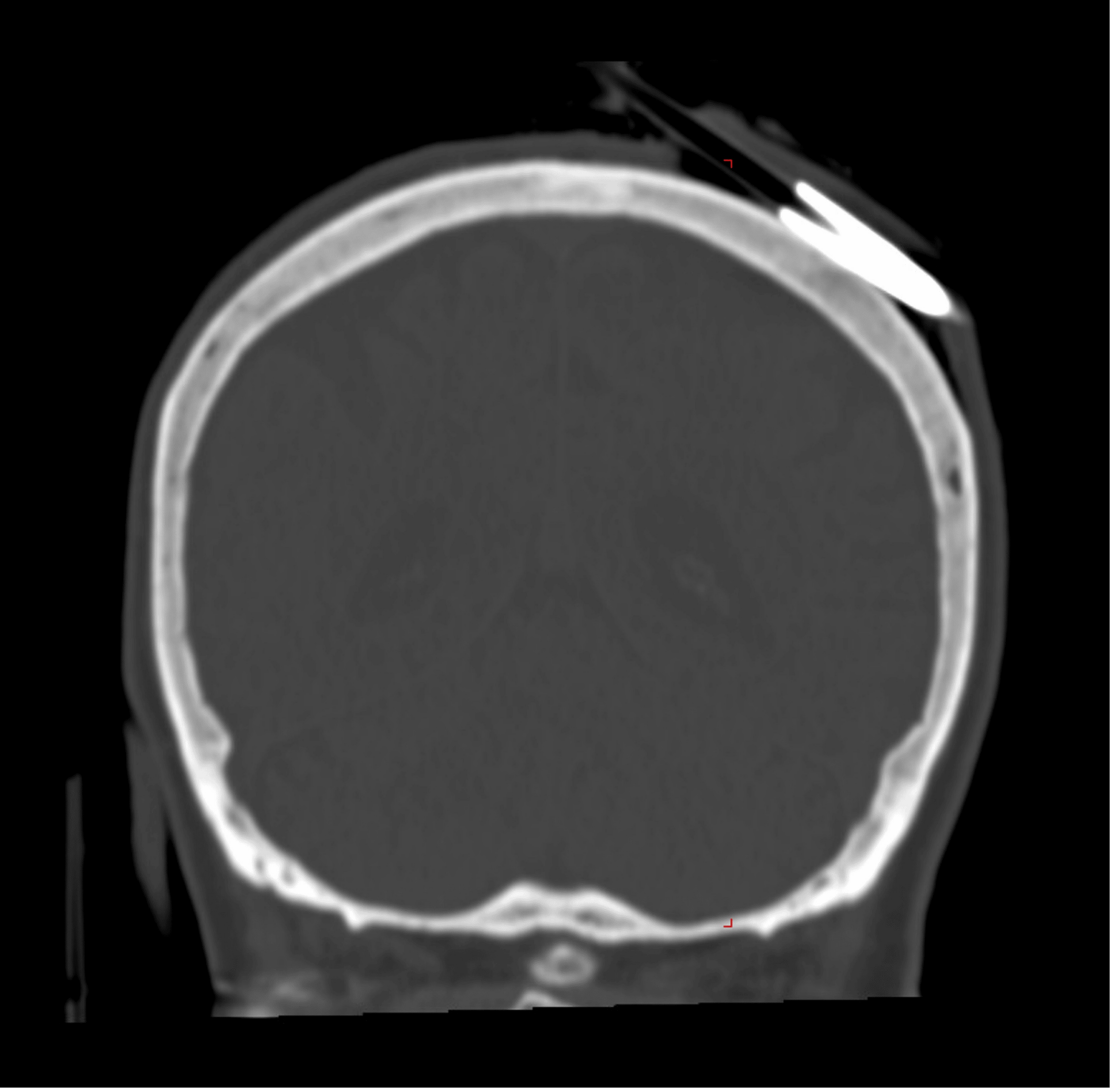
Coronal head CT. Soft tissue injury over the left parietal region with the arrow tip in contact with the external cortical bone, without evidence of intracranial involvement. CT: computed tomography
